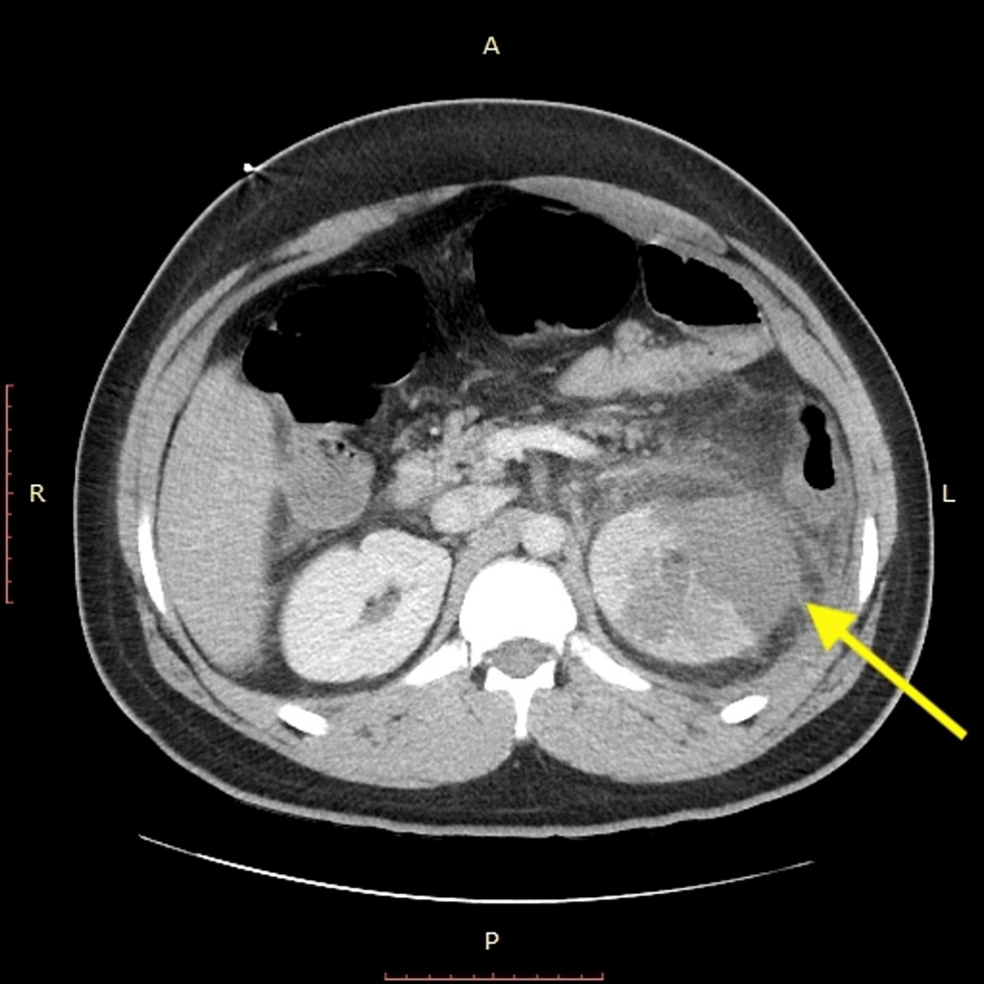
Abdominal computed tomography scan with contrast showing left renal infarction.
